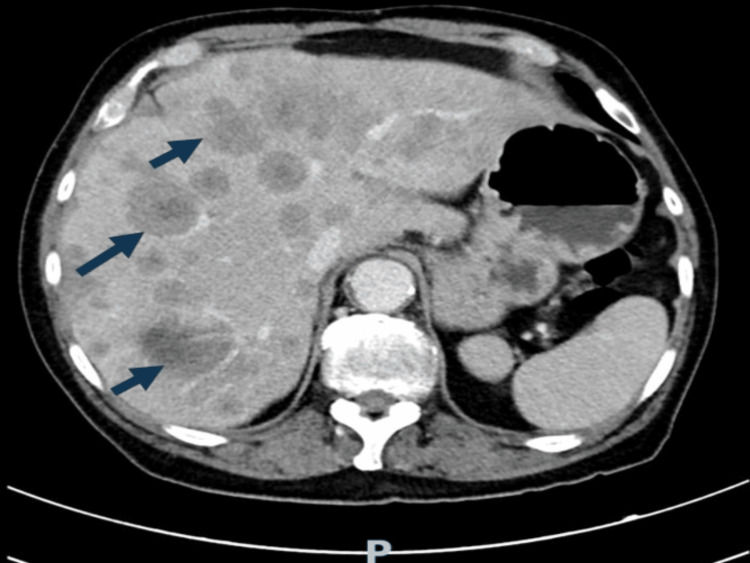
Abdominal CT scan revealing an enlarged liver with multiple heterogeneous hypodense lesions consistent with extensive hepatic metastasis.
